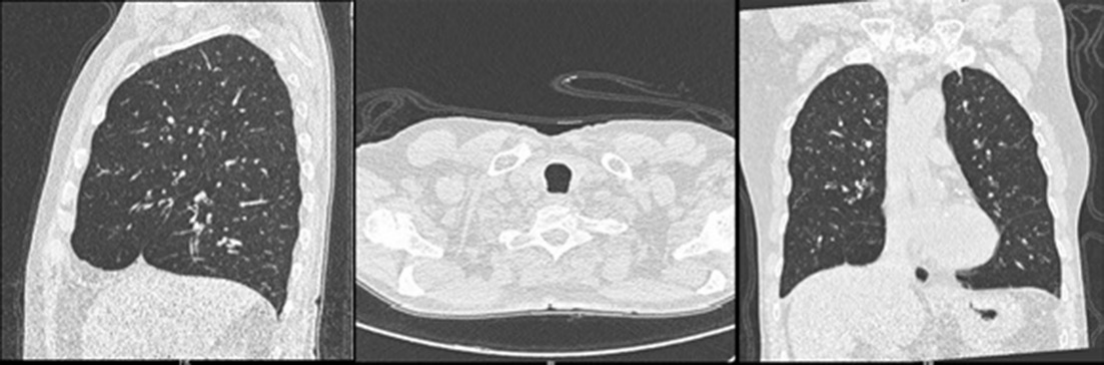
CT chest showed centriacinar emphysematous changes involving the bilateral lung parenchyma, predominantly the bilateral upper lobe parenchyma. Central tubular bronchiectasis with bronchial wall thickening is seen involving the segmental and lobar bronchi of the right middle and lower lobe, and lower lobe of the left lung parenchyma.
